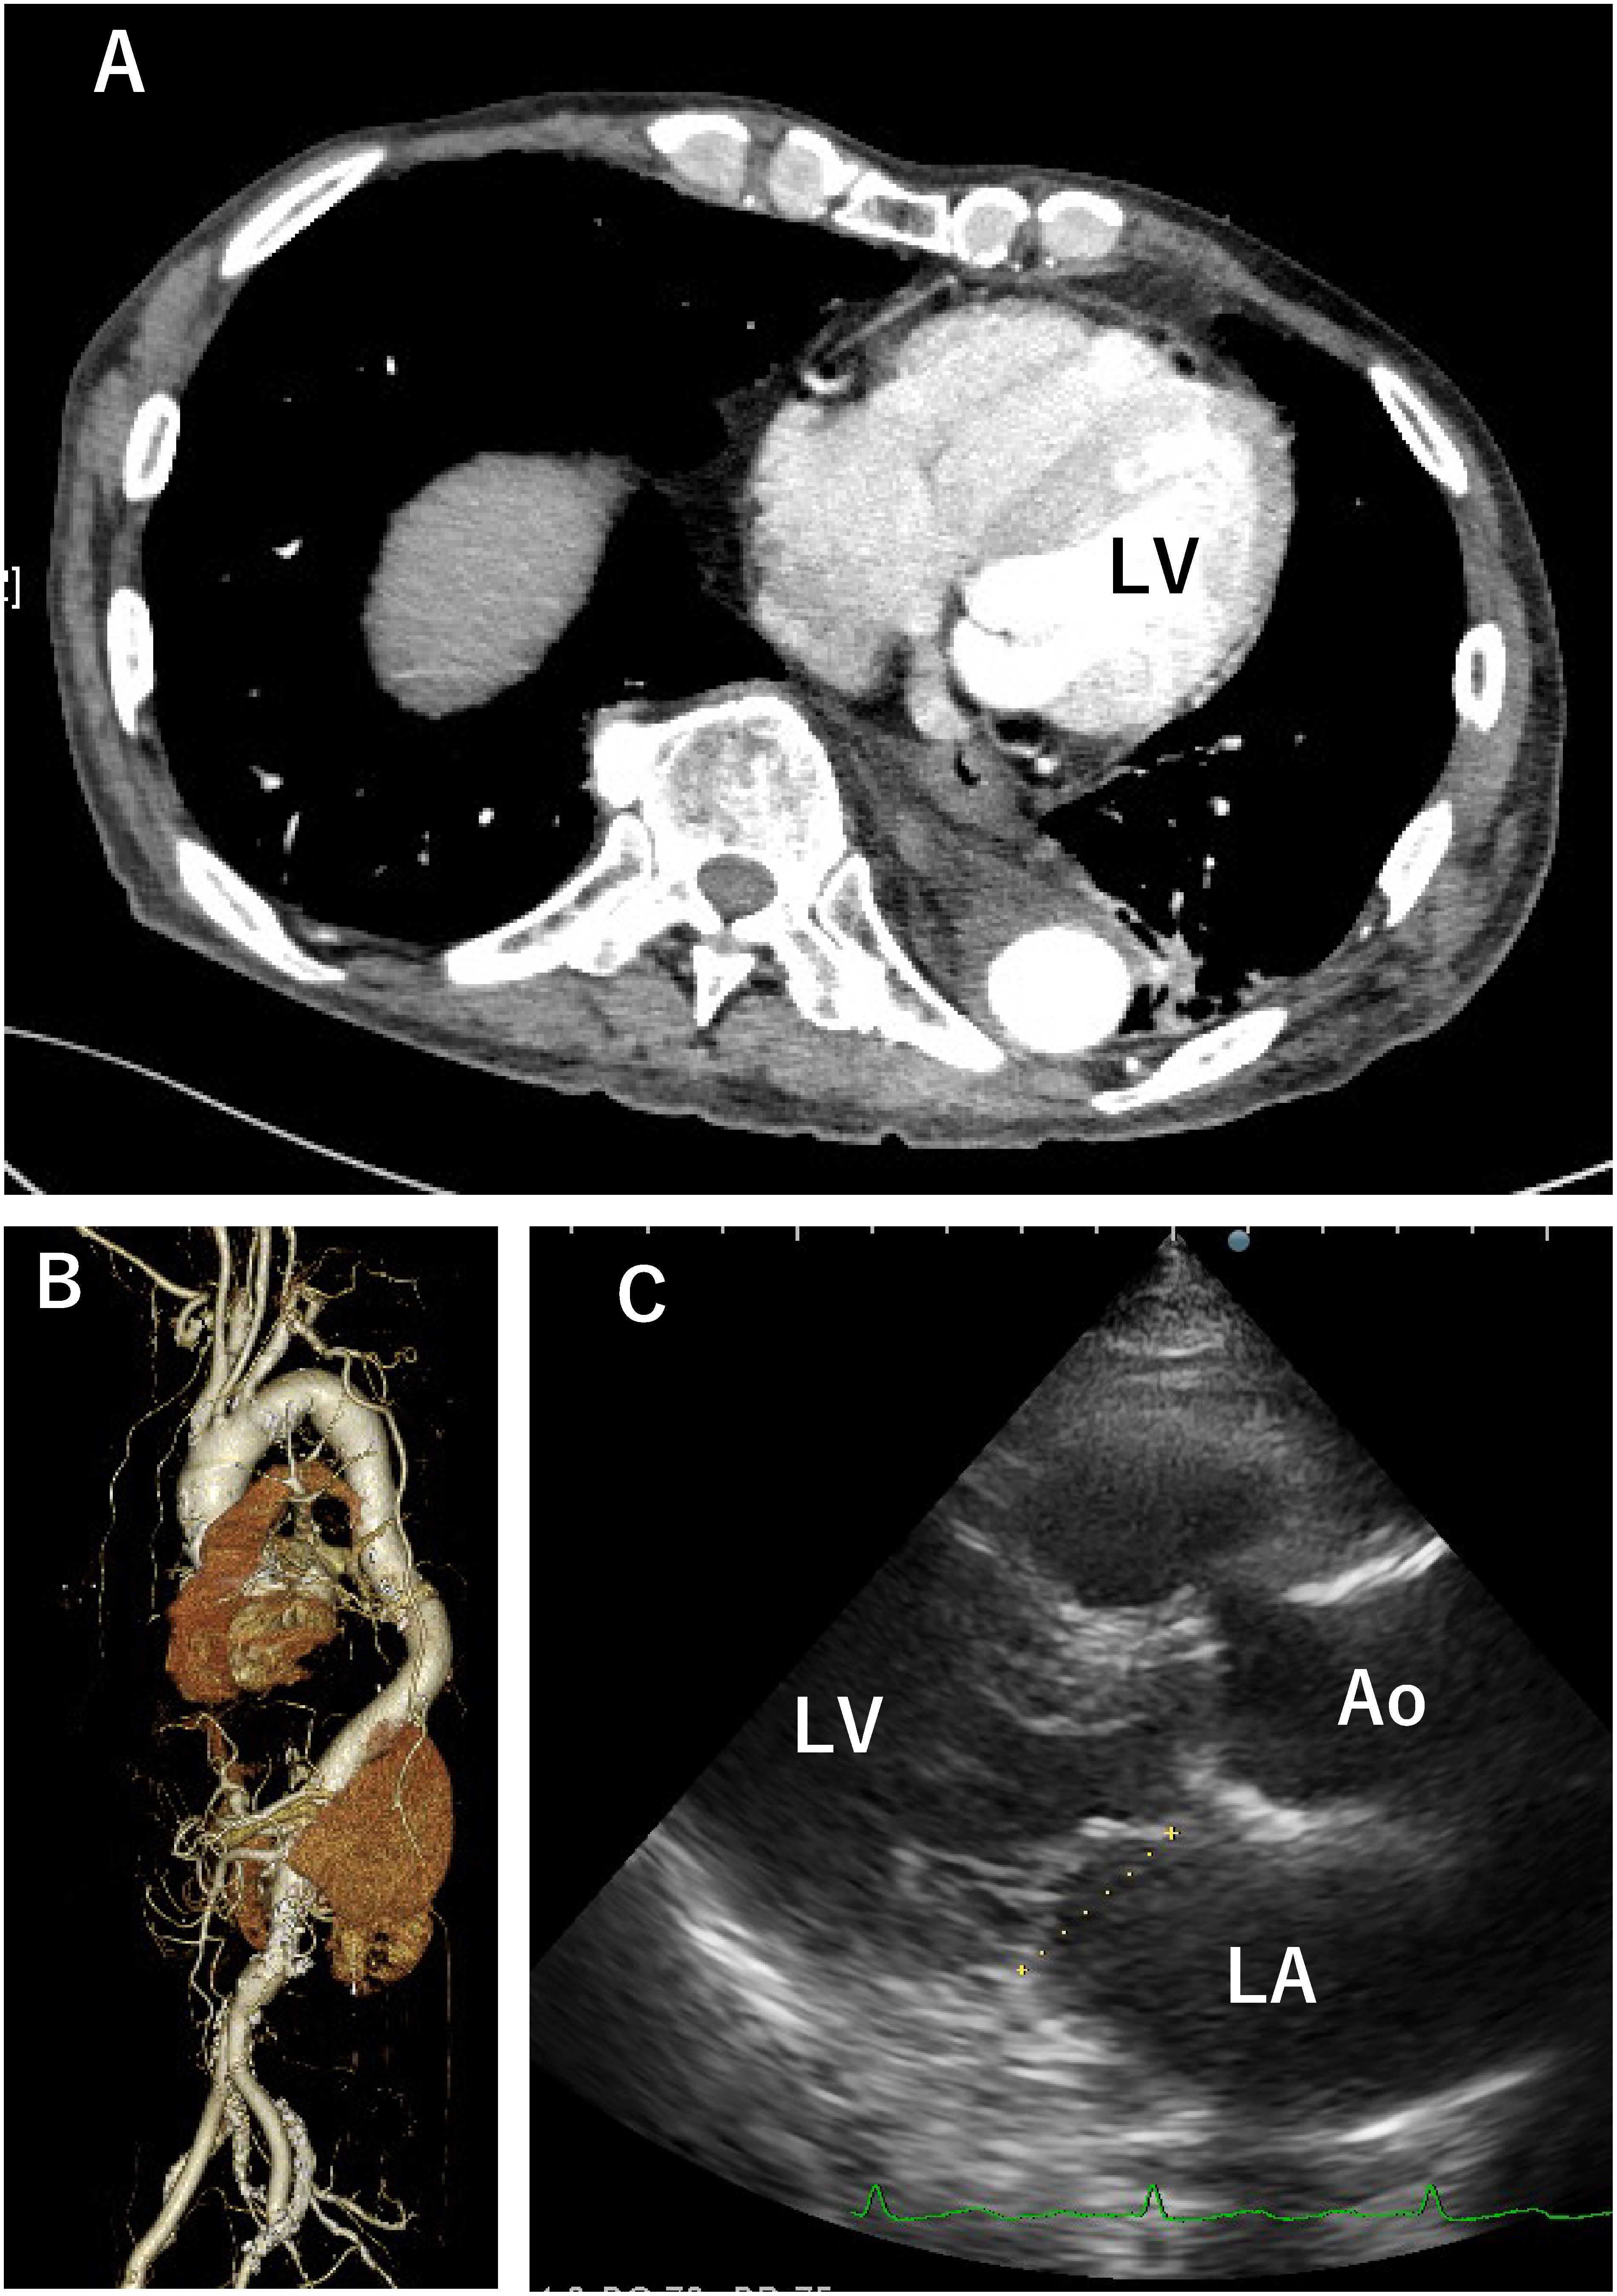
Fig. 3 Postoperative contrast-enhanced computed tomography (A, B) and transthoracic echocardiography (C) showing a well-repaired thoracoabdominal aorta and the LA and LV released from the compression of aortic aneurysm.
